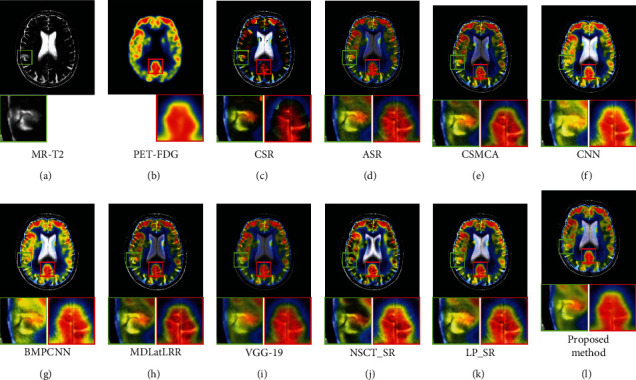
Comparison of the fusion results in the third set images. (a) MR-T2. (b) PET-FDG. (c) CSR. (d) ASR. (e) CSMCA. (f) CNN. (g) BMPCNN. (h) MDLatLRR. (i) VGG-19. (j) NSCT_SR. (k) LP_SR. (l) Proposed method.
